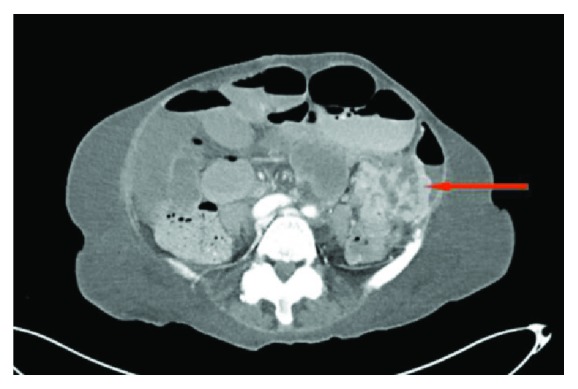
CT scan of the abdomen. Image shows dilated small bowel loops with air fluid level and small bowel obstruction.
